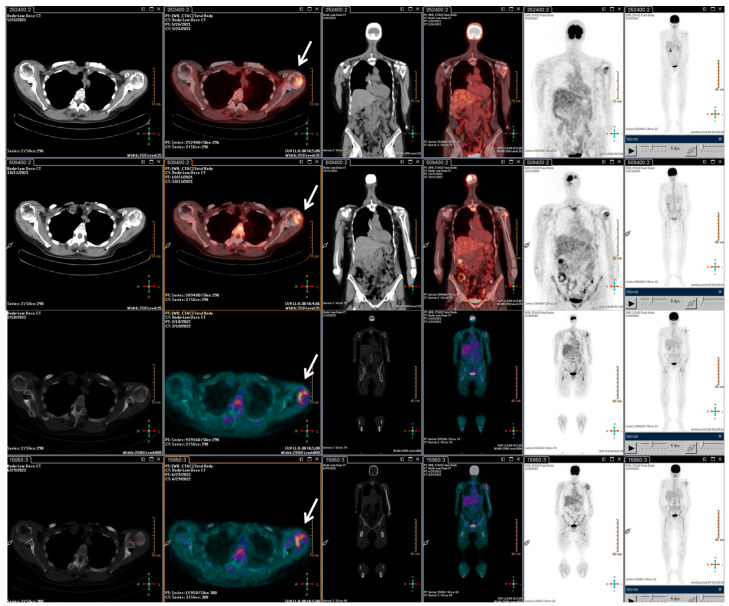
F-18 FDG PET/CT performed after the radiotherapy retreatment. The main tumour lesions are indicated by white arrows.
